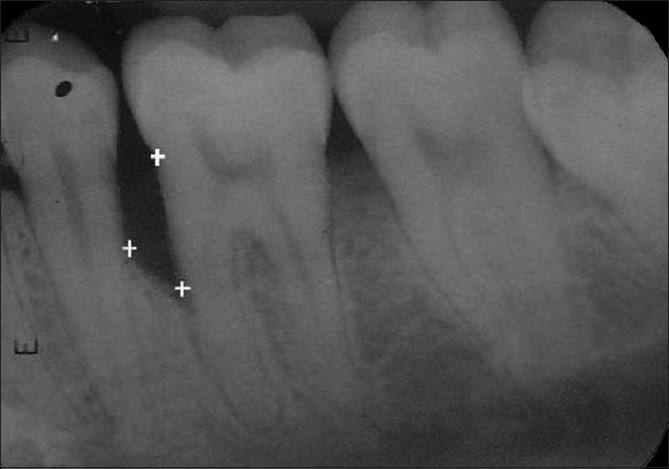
Method of recording linear radiographic measurements
